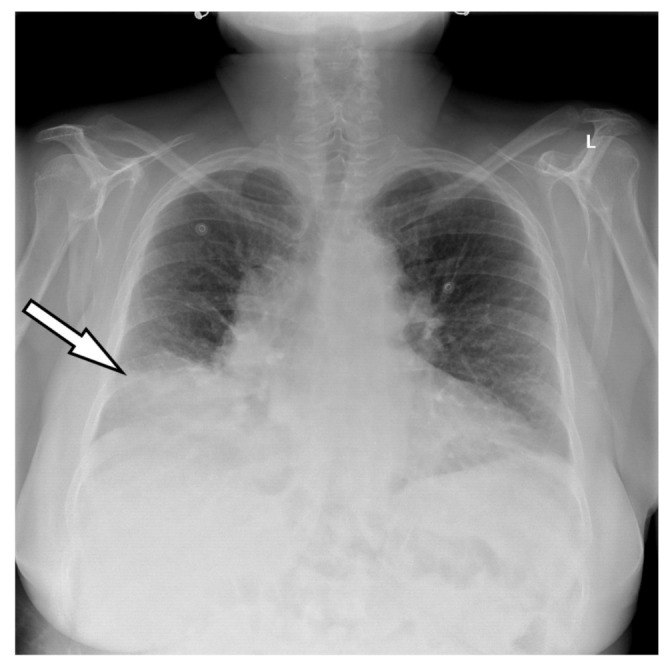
Posteroanterior chest X-ray: a right lower pulmonary lobe consolidation.
